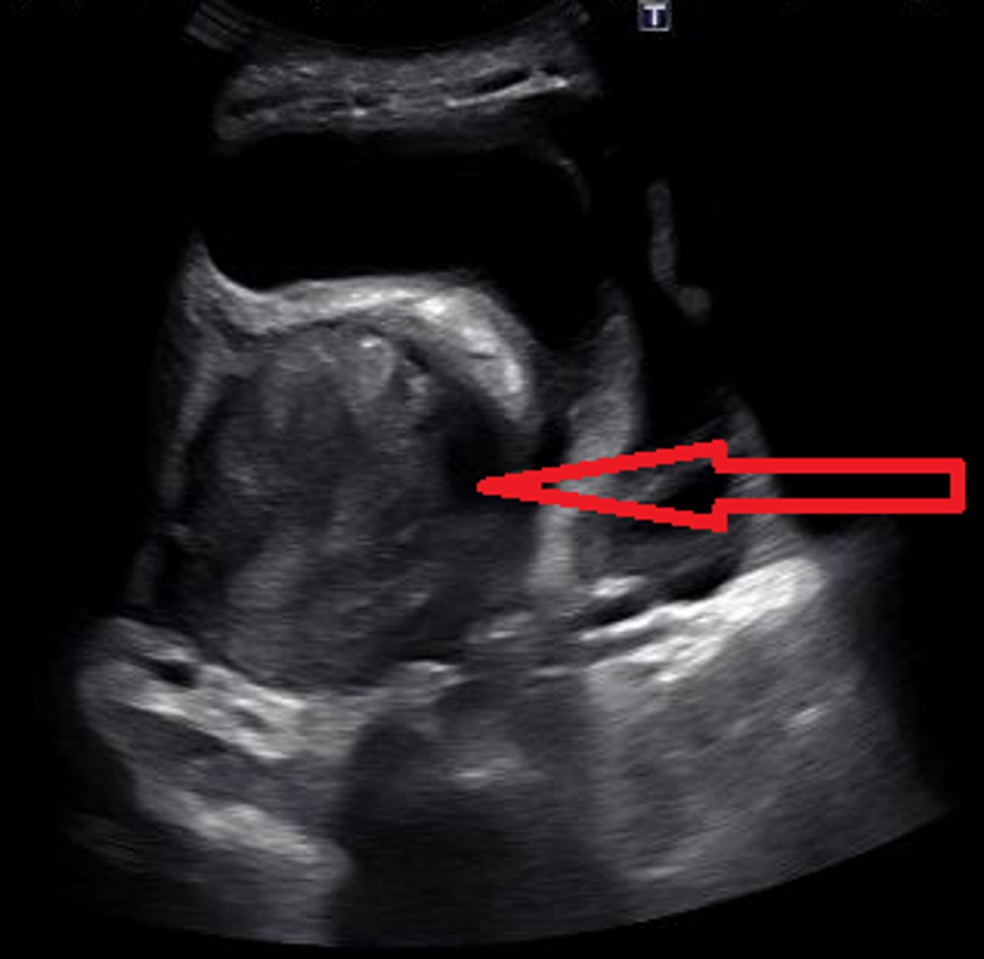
Transvaginal ultrasound showing a large septate cystic lesion in the left adnexa (red arrow), which has an appearance consistent with endometrioma T: transvaginal.
